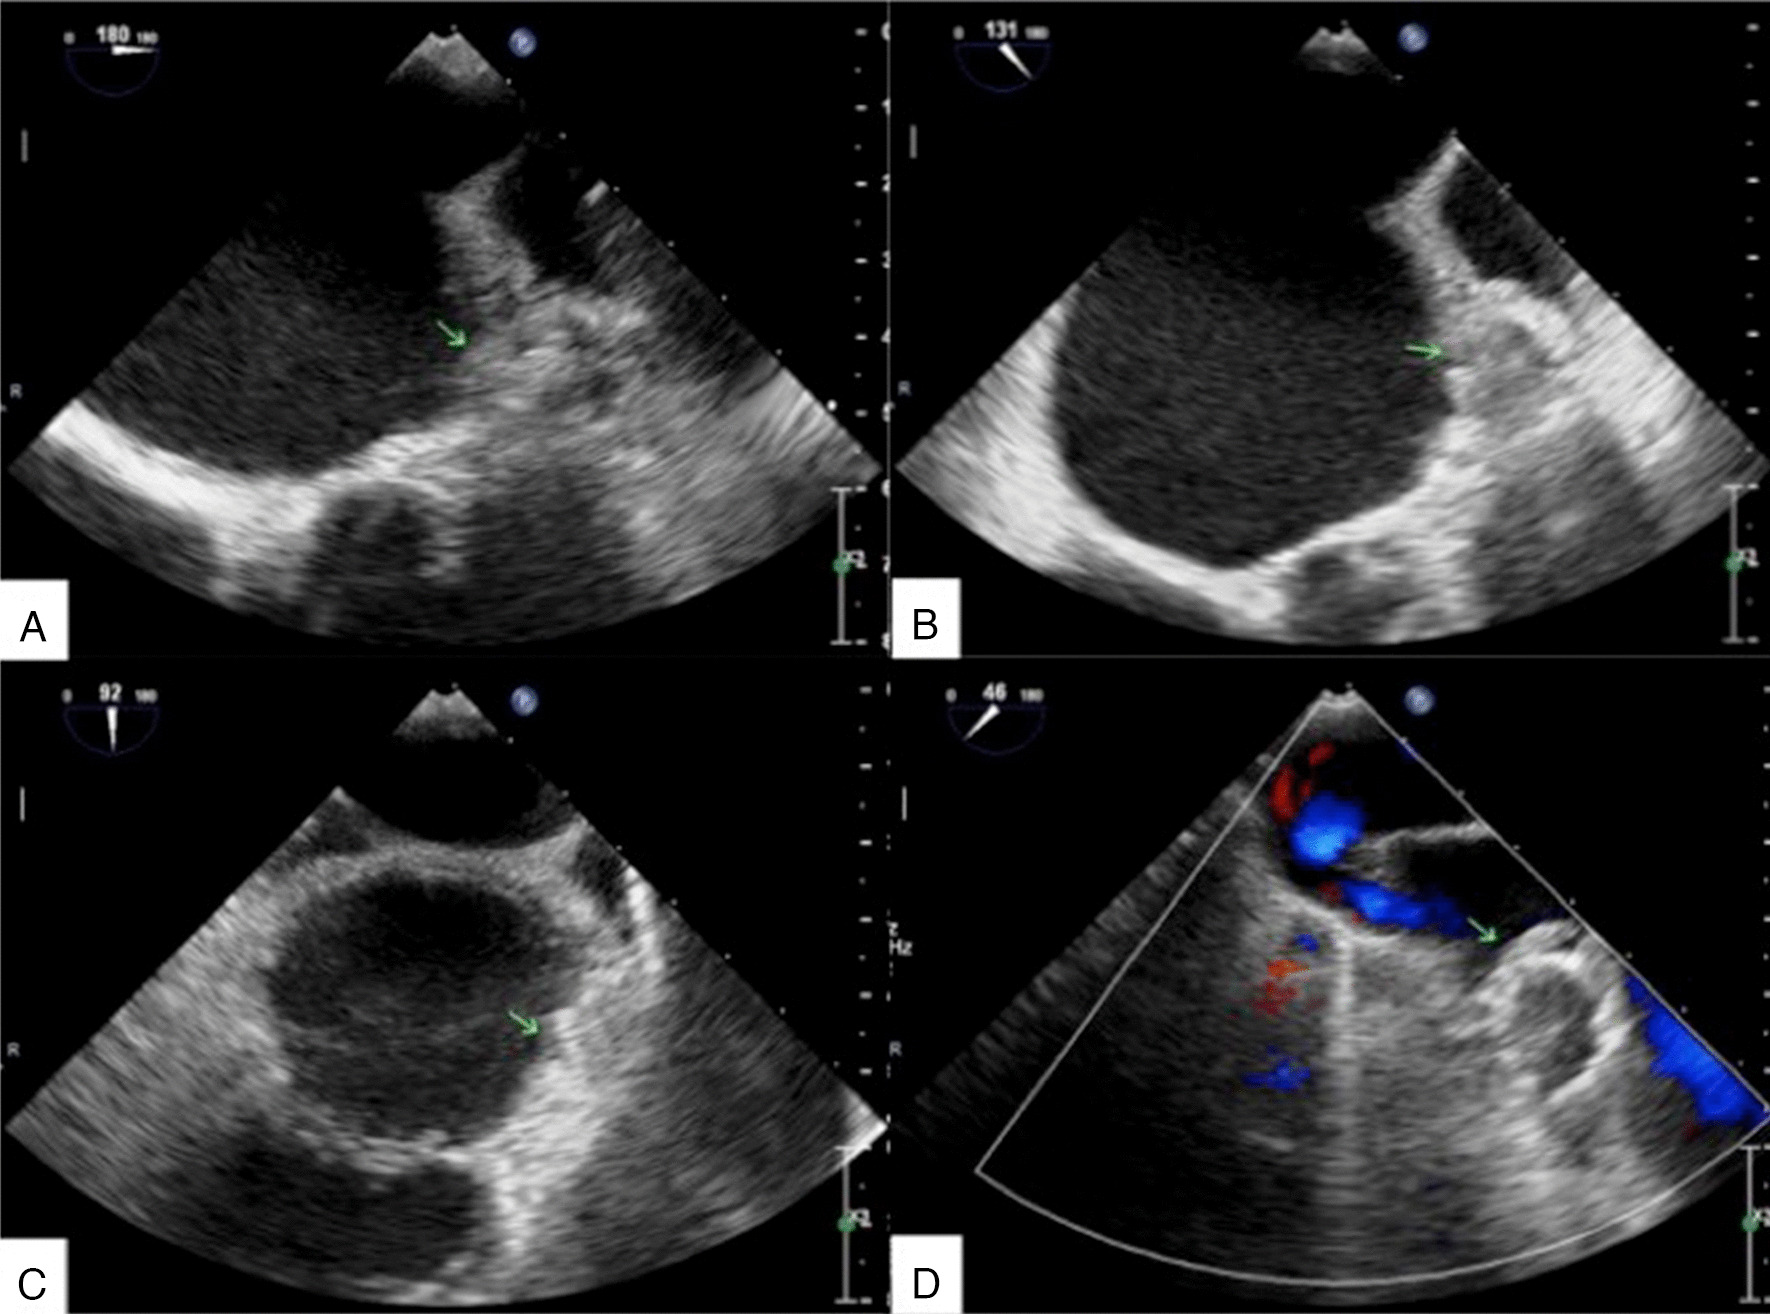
Verification at different angles after Watchman device implantation. Transesophageal echography (TTE) showed that the device was implanted in a favorable position without residual leakage. Following the PASS principle (position, anchor, seal, and size), the stability was tested after the device was implanted. The ratio of compression was 20–23%
